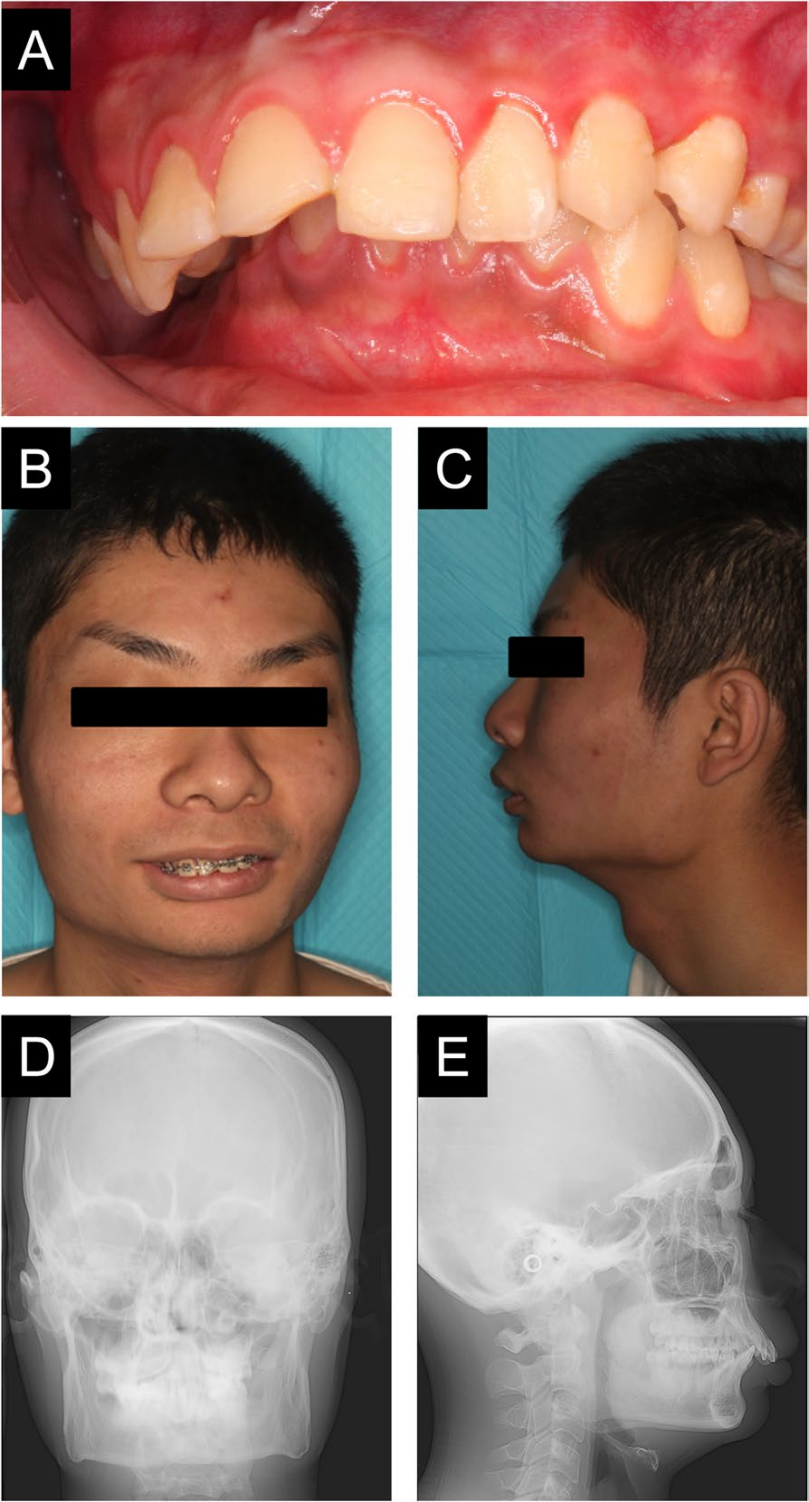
Preoperative photographs and radiographs. Intraoral photographs at the time of tooth extraction (A). Frontal view (B) and lateral view (C) on facial photographs before maxillary osteotomy. Frontal cephalogram (D) and lateral cephalogram (E) showing radiographic findings at the time of tooth extraction
